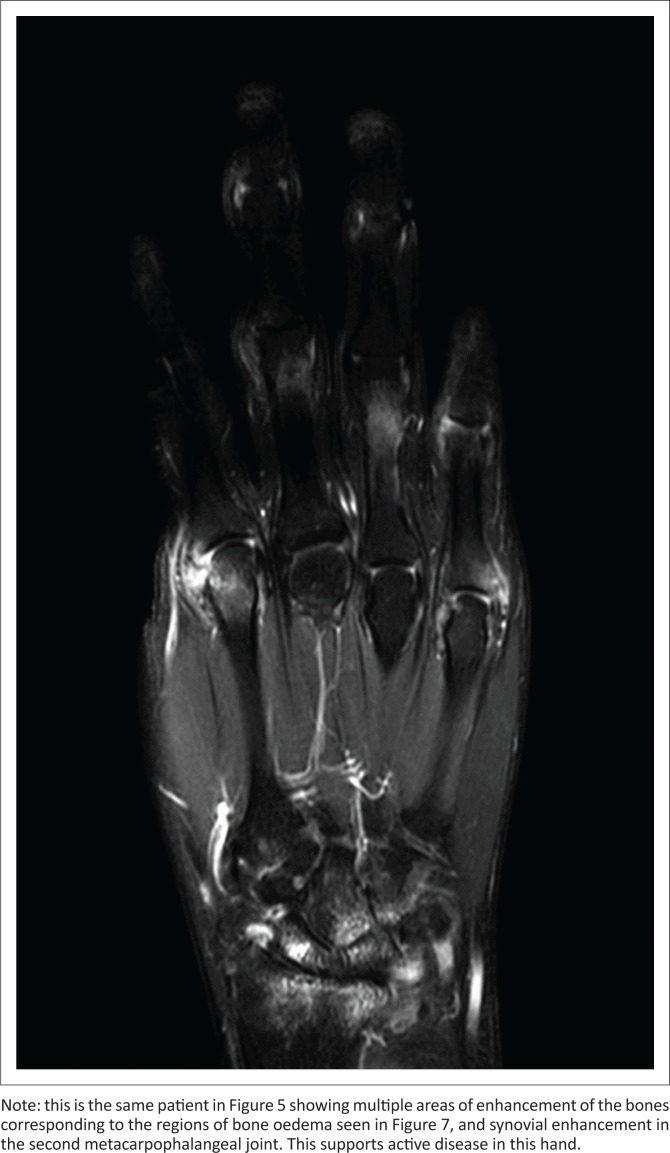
Post-contrast fat-suppressed T1-weighted coronal magnetic resonance imaging.
